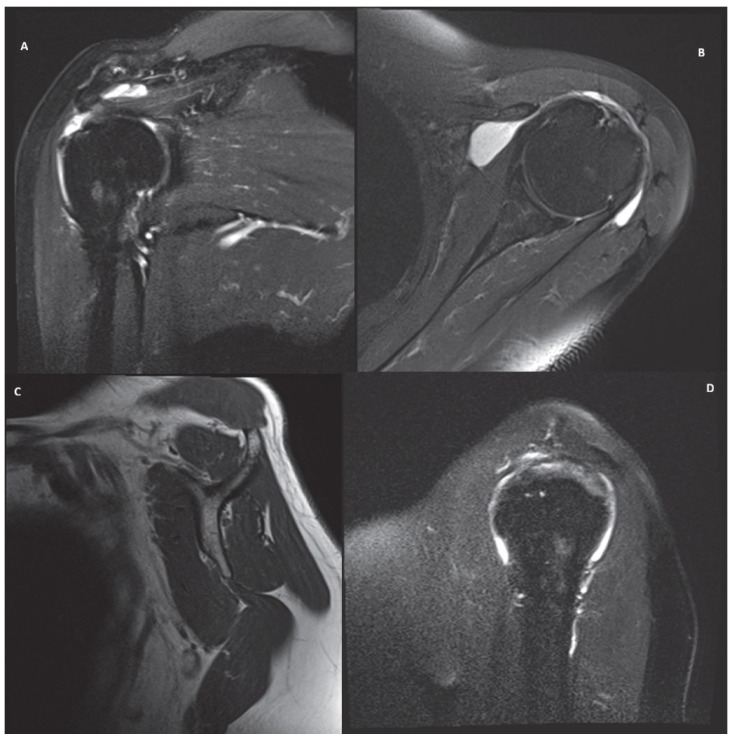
MRI images of a partial bursal supraspinatus tear in coronal (A), axial (B) and sagittal (C,D) view.
